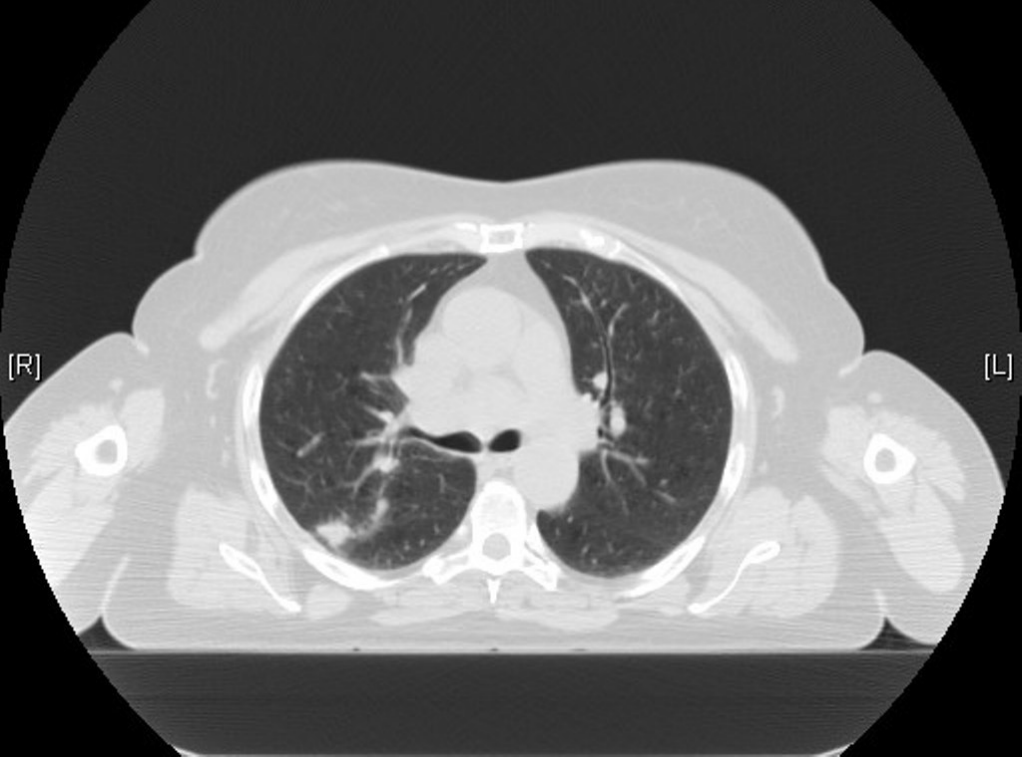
CT scan of the chest from 2004 demonstrating nodular opacity in the posterior aspect of right upper lobe
